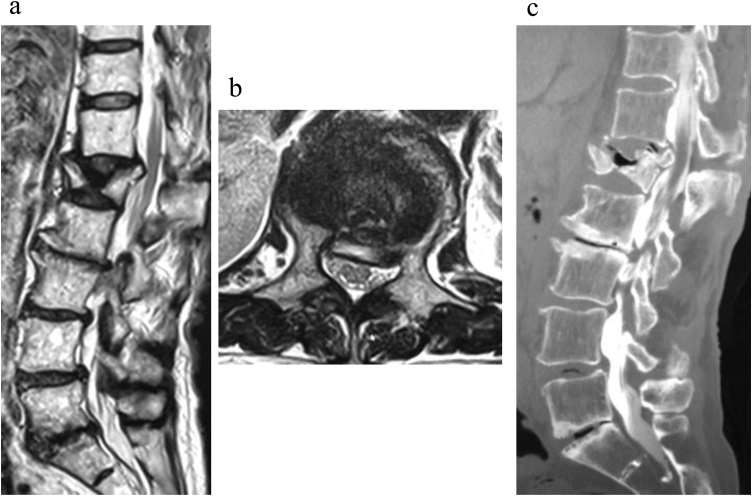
MRI and CT myelogram of the thoracolumbar spine. Views: (a) MRI of T2-weighted sagittal image, (b) MRI of T2-weighted axial image at L1, and (c) Sagittal image reconstruction of CT myelogram.
